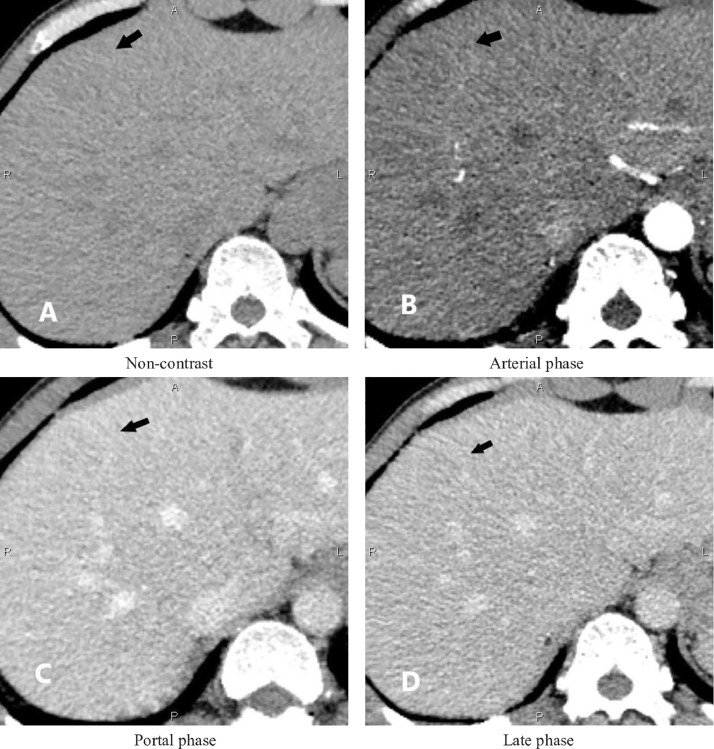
Contrast-enhanced CT reveals multiple hypoattenuating lesions (A) without enhancement in the arterial phase (B), the portal phase (C), and the late phase (D) (arrow).
